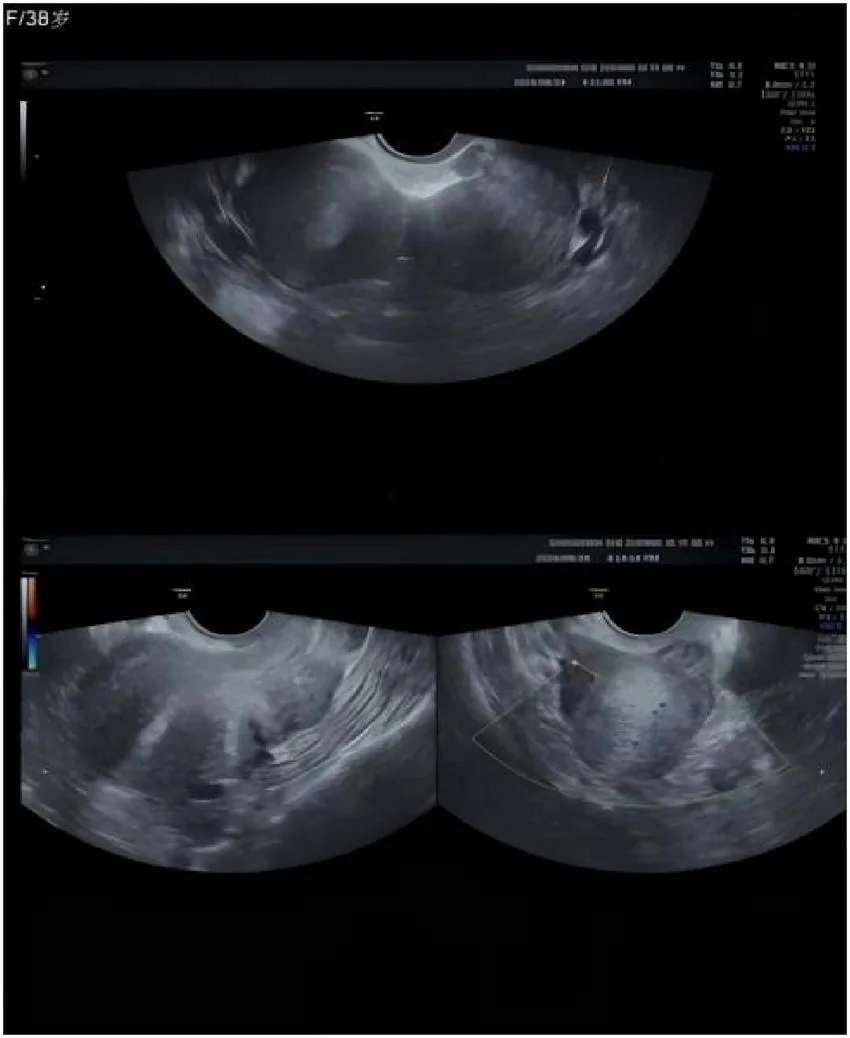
Preoperative transvaginal three-dimensional ultrasound showing uterus didelphys (single-vagina type) complicated by bilateral diffuse adenomyosis.
